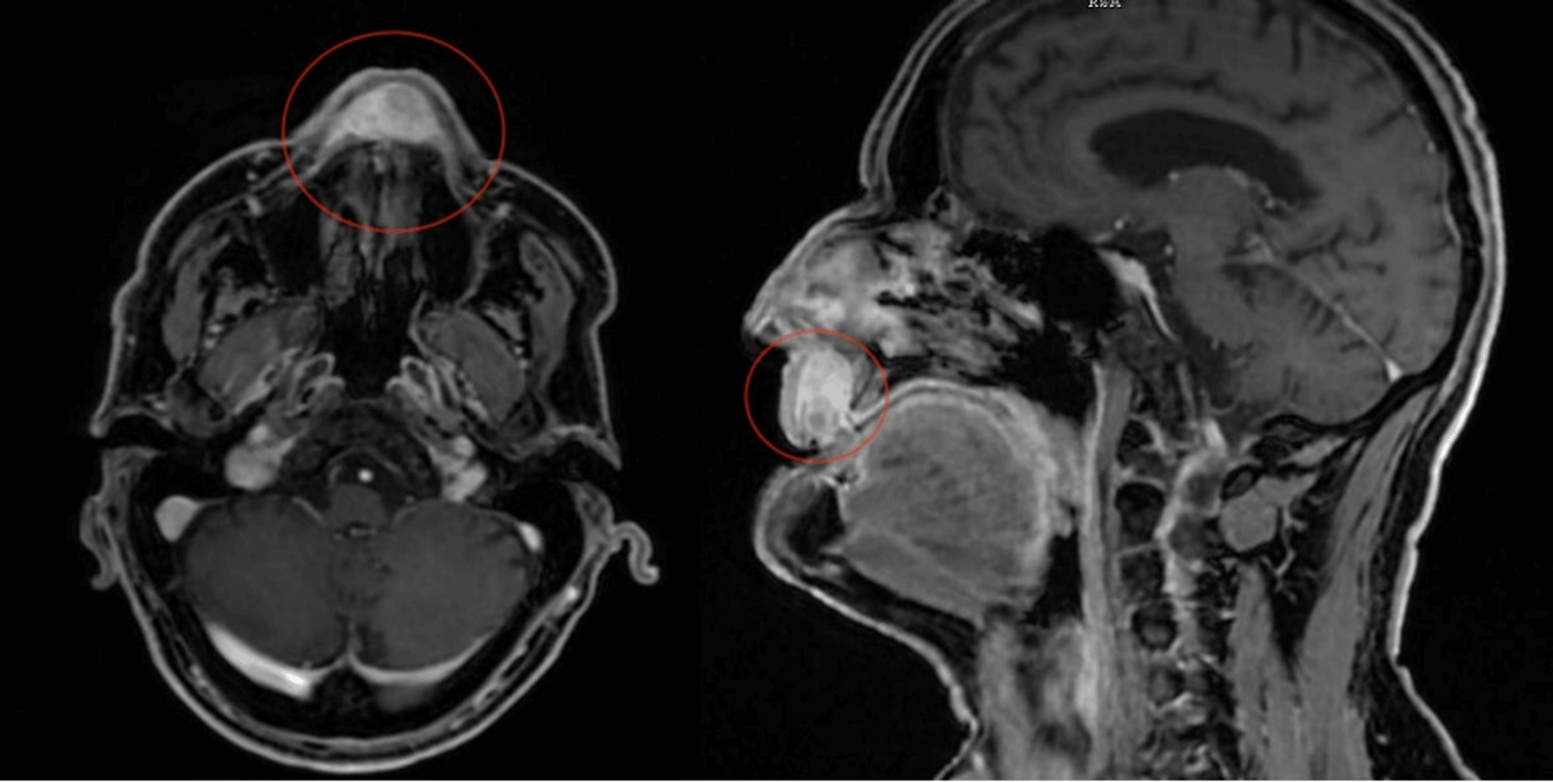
MRI scan (T1-weighted post-contrast sequence) showing the lesion in the surrounding soft tissue of the upper lip Left: axial view. Right: sagittal view. The tumor is indicated by a red circle, with its borders limited to the soft tissues of the upper lip.
